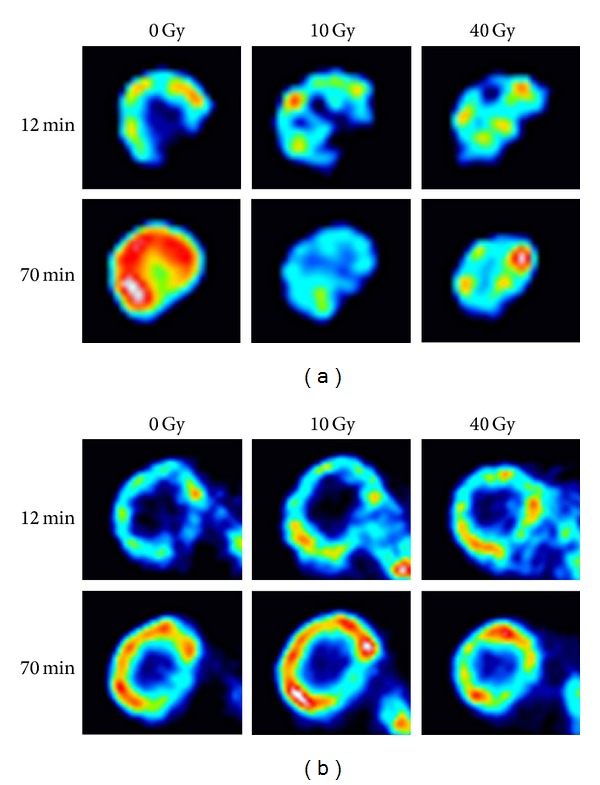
Comparison of FDG-PET changes over time between locally controlled versus uncontrolled tumors. The images represent raw tumor activities with window and level automatically set to reflect all activity values for the images at 12 minutes. The same settings are then applied to each corresponding image at 70 minutes from the same dynamic PET scan. (a) A locally controlled tumor. (b) A locally uncontrolled tumor. PET images prior to radiotherapy (0 Gy), at week 1 (10 Gy) and at week 4, (40 Gy) are shown as tumor activity at 12 min and 70 min (after injection).
